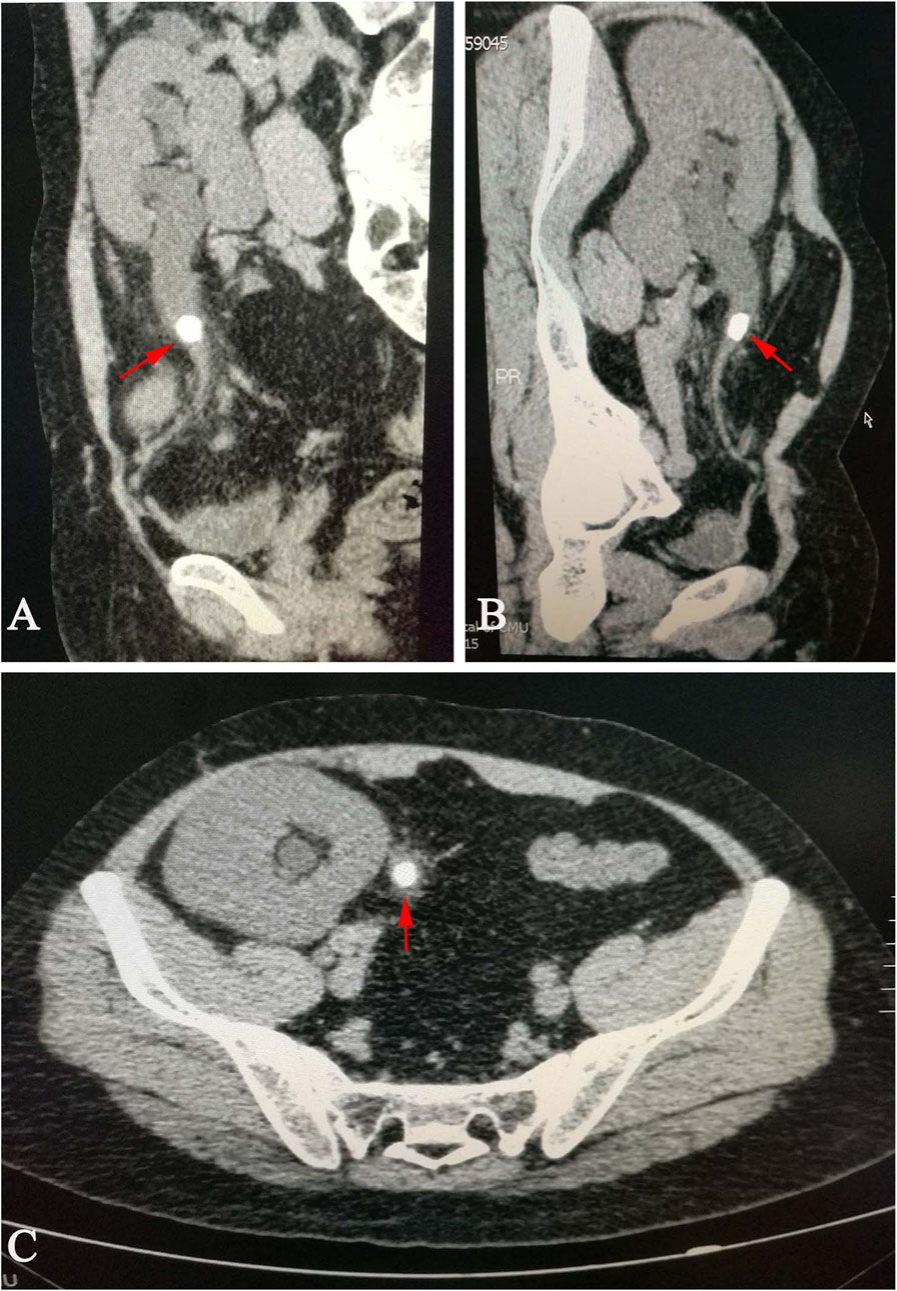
Preoperative CT scan showing a proximal ureteral calculus with severe hydronephrosis and extension of the ureter. a Longitudinal plane left view, b longitudinal plane right view, c transverse plane
